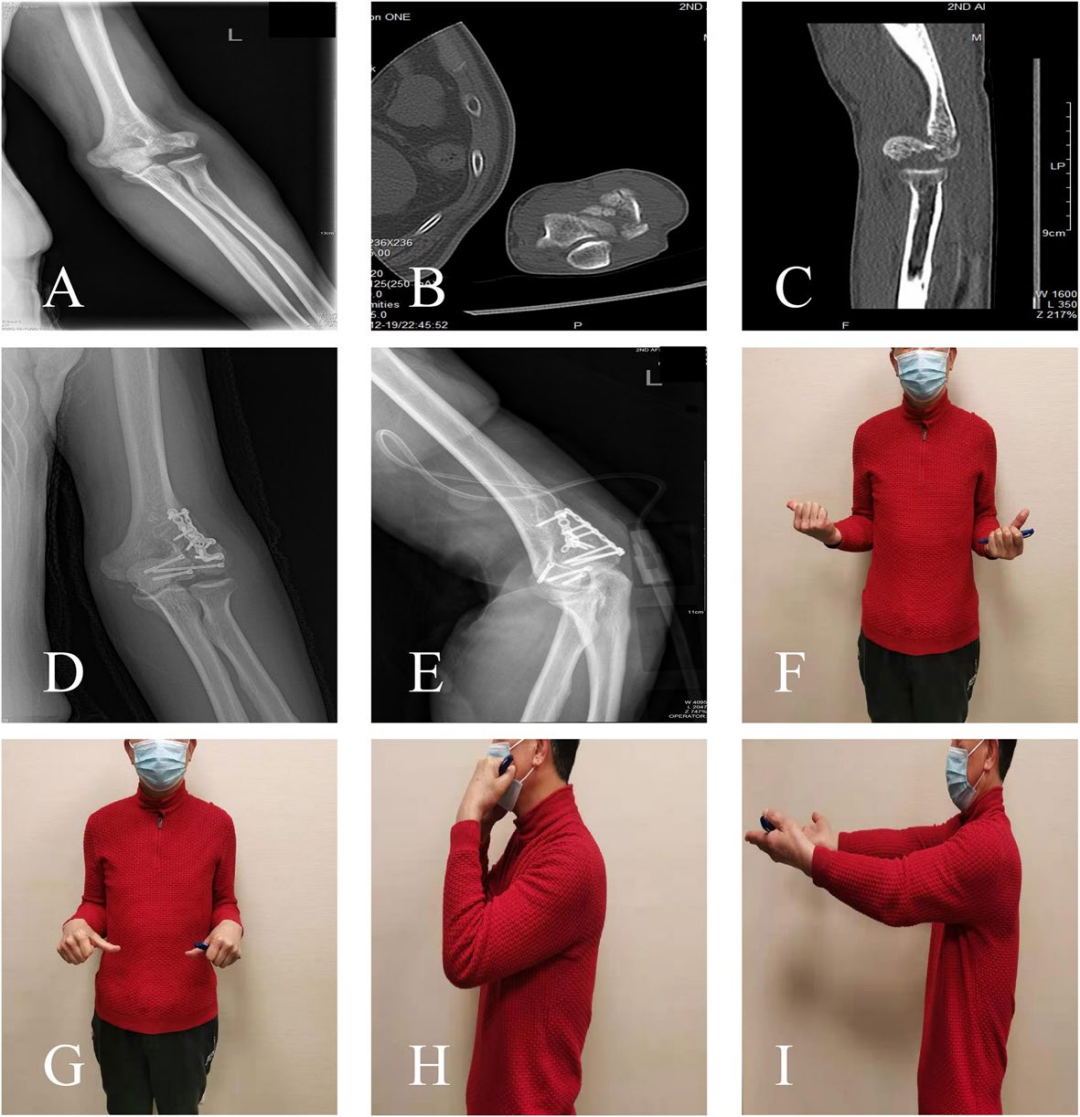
A type IIIB capitellum fracture in a 68-year-old male patient. A Preoperative anteroposterior radiograph and B, C CT scan. D, E X-ray plain film at 12 months after surgery. H–K Mild limitation of elbow flexion and pronation at 12 months of follow-up; the Mayo Elbow Performance Index is 90 points
